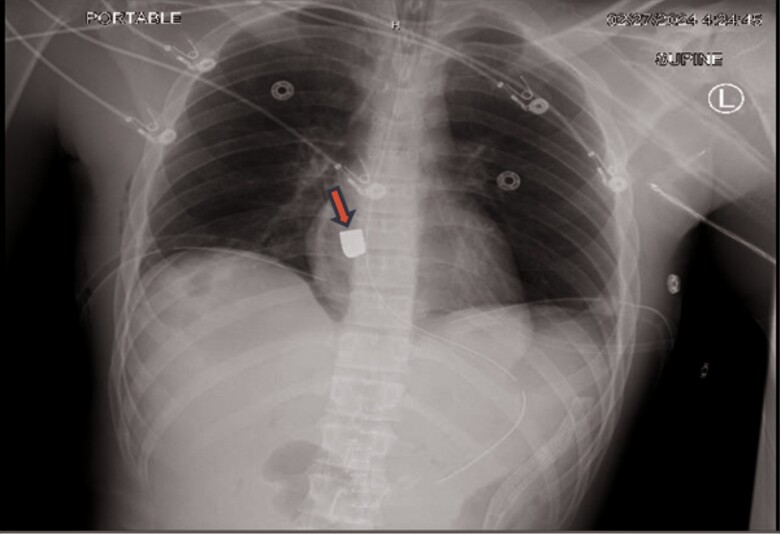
Repeat chest X-ray demonstrating a change in the bullet’s location and orientation.
